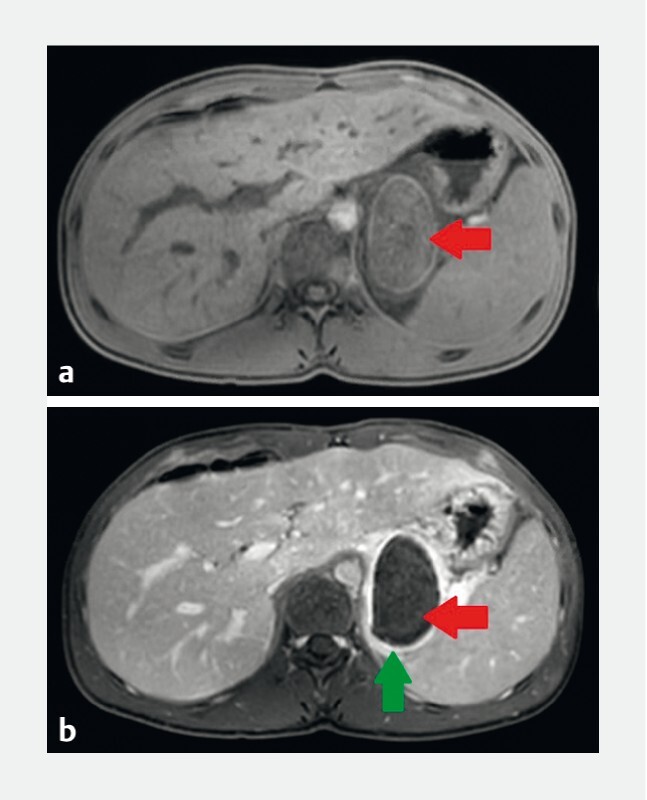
Abdominal magnetic resonance imaging showing a retro-gastric cystic lymphangioma.
